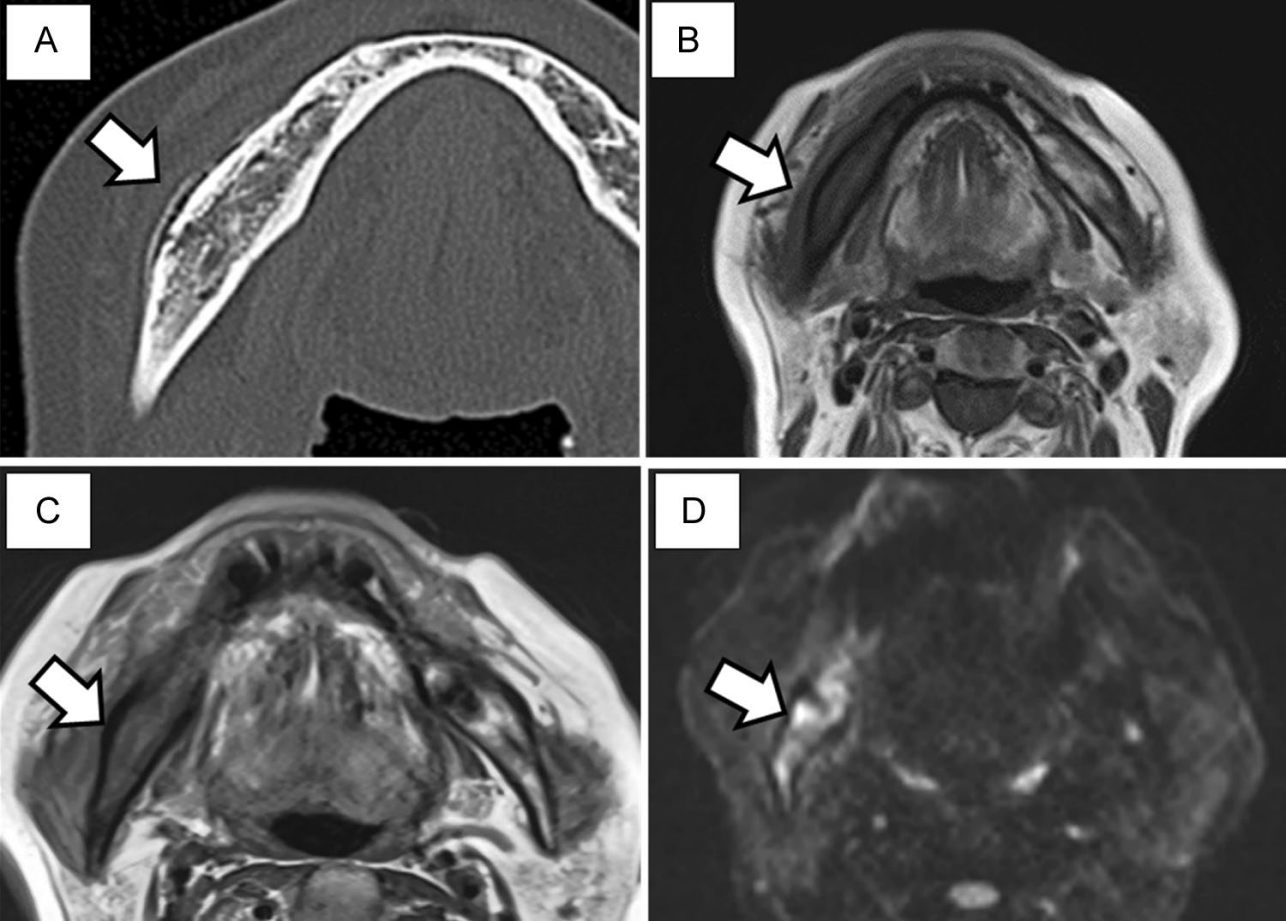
Imaging findings after 4 months. (A) An extensive gap-type periosteal reaction is observed on the buccal side of the right mandible. Magnetic resonance imaging. (B) Bone marrow signal of the right mandible is reduced in T1-weighted imaging. Low signal continuity is observed in the cortical bone. (C) A high signal in the soft tissue around the right mandible and decreased bone marrow signal are observed in T2WI images. (D) A high signal is observed from the right mandible to the condyle and in the surrounding soft tissue on T2 short tau inversion recovery imaging.
